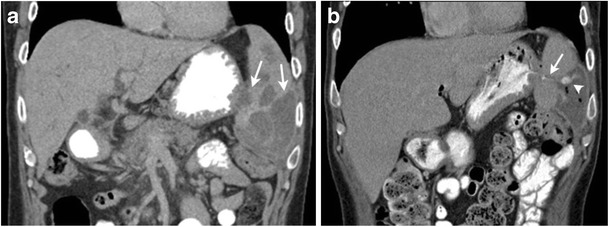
A 49-year-old male with relapsed HL with splenic lesions treated with second-line chemotherapy. a Coronal contrast-enhanced CT image shows splenomegaly with multiple low-attenuation splenic lesions (*arrows*). b Coronal contrast-enhanced CT image 4 months after treatment with second-line chemotherapy demonstrates communication between the stomach and spleen (*arrow*) with the presence of hyperdense oral contrast material (*arrowhead*) and air specs in a low-attenuation splenic lesion suggestive of gastrosplenic fistula
